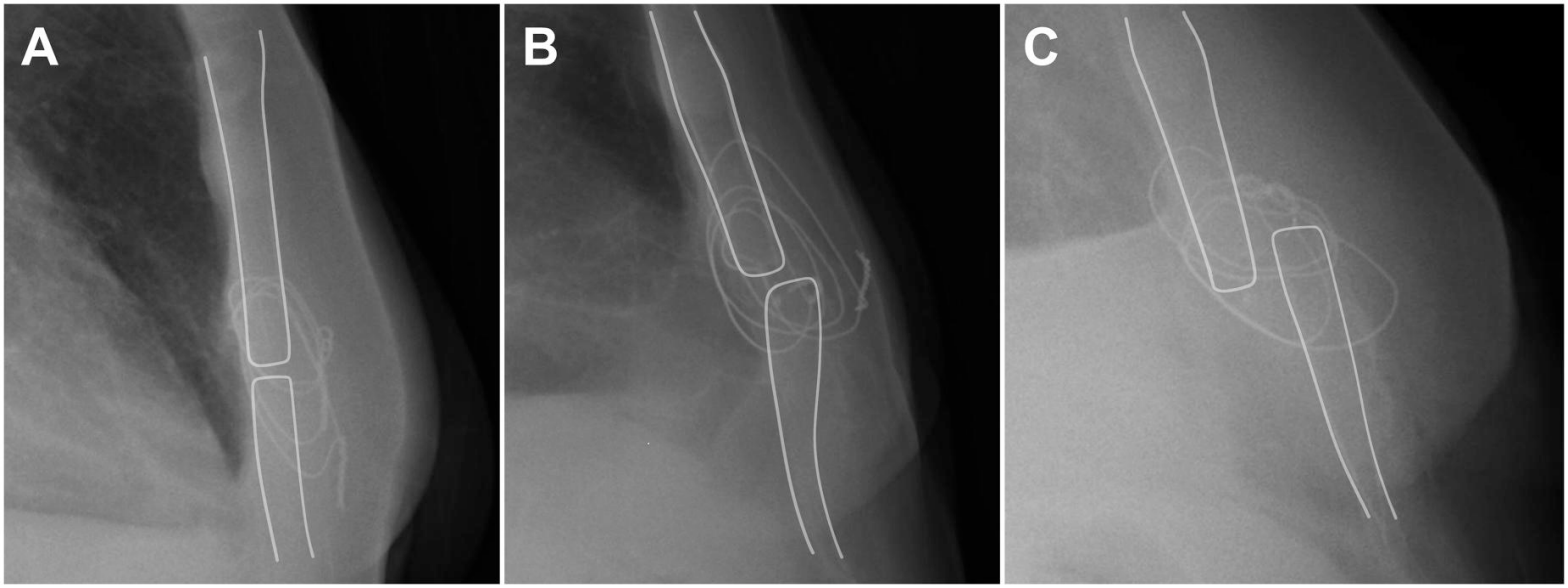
Lateral chest radiographs of three patients demonstrating different degrees of sternal alignment, according to the classification of Koster *et al*.^9^ (A) Normal alignment; (B) override; (C) separation. The sternal borders are highlighted with white lines to aid visualization.
